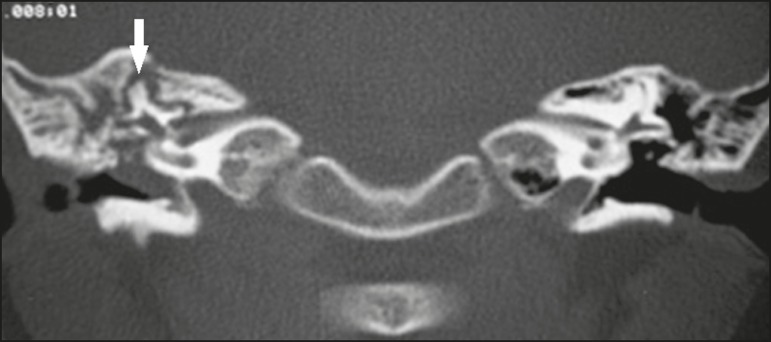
Osteomyelitis-complicated otomastoiditis. Coronal CT, with a bone
